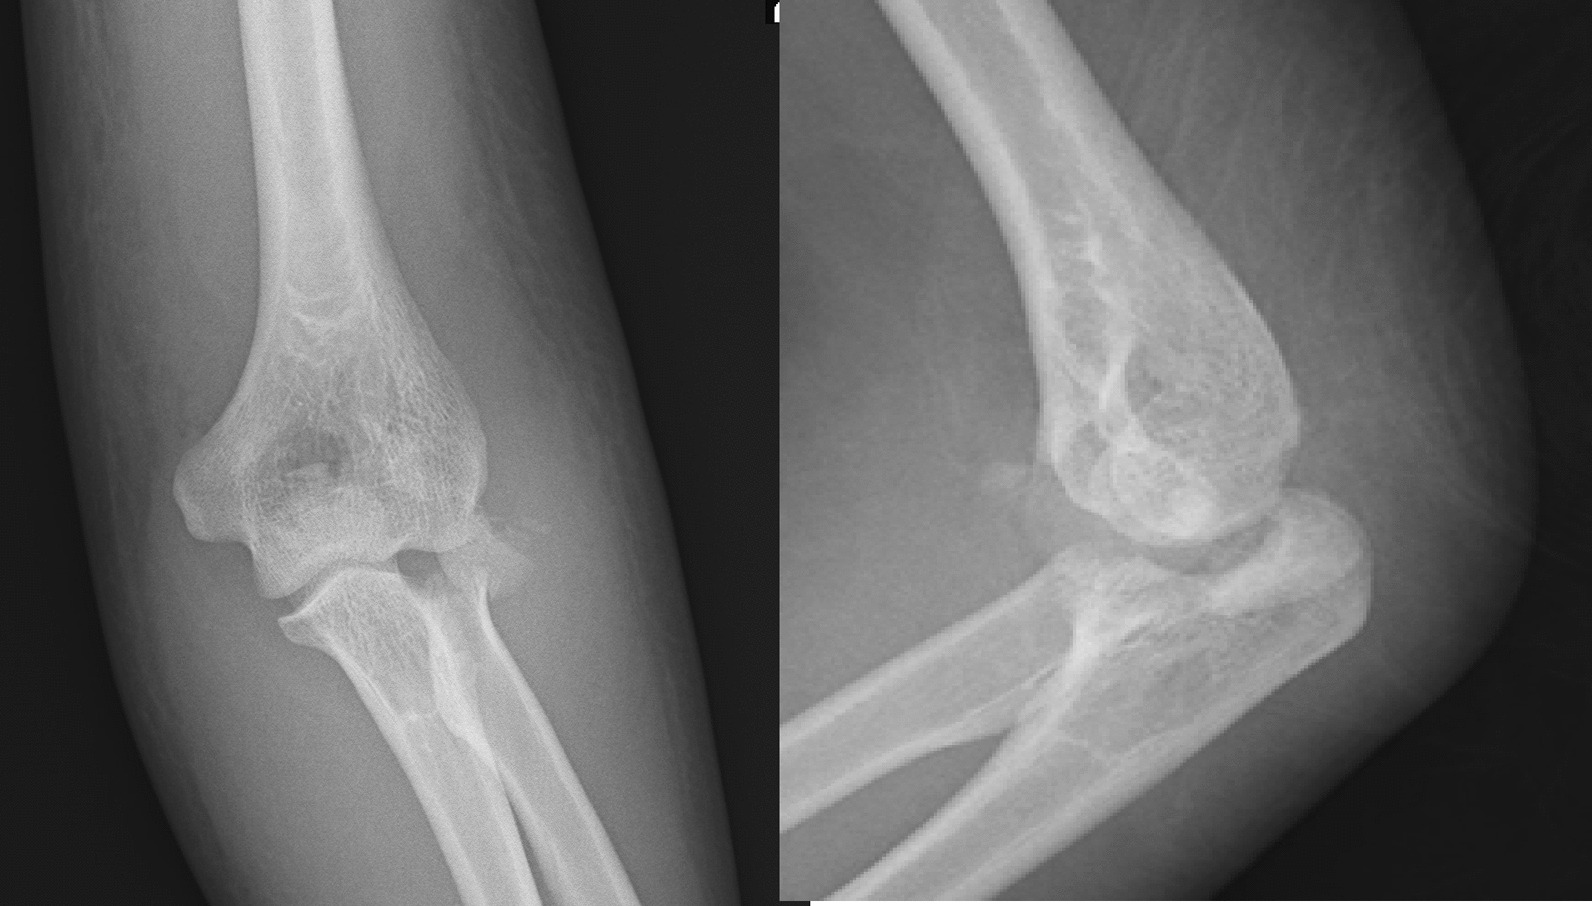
Post-reduction anteroposterior and lateral view X-ray of the left elbow; the anteroposterior view reveals the presence of a displaced radial head fracture, while the lateral view indicates the occurrence of a coronoid fracture
